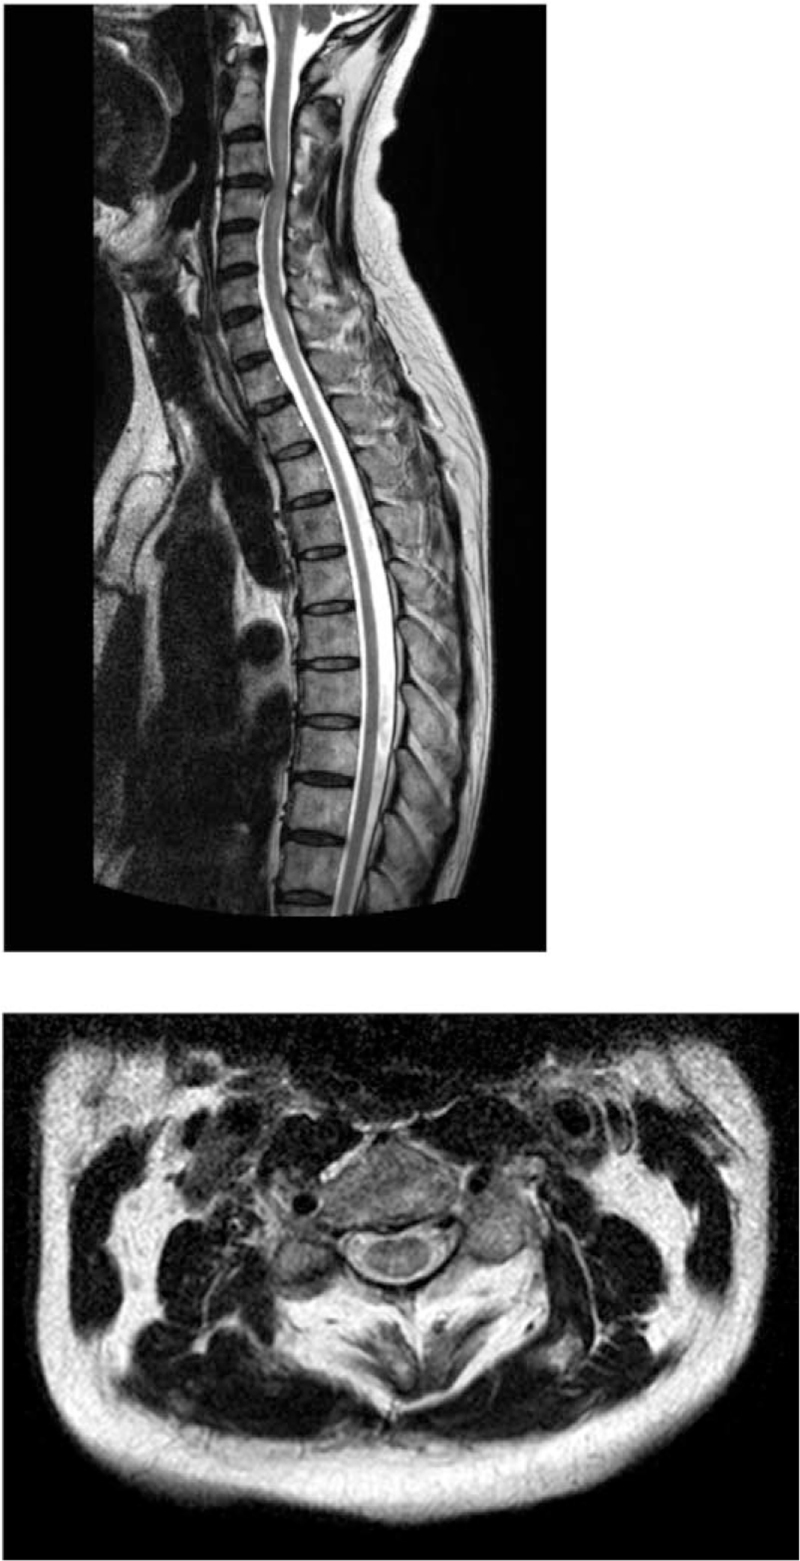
Contrast-enhanced cervical thoracic spine MRI A very faint hyperintensity is suspected on T2WI from the cervical spinal cord to a part of the middle thoracic spinal cord (both lateral cords to part of the posterior cord). Compared to MRI 6 months earlier, this finding is more evident in the cervical cord. No abnormal contrast findings were observed. MRI = magnetic resonance imaging, T2WI = T2-weighted imaging.
